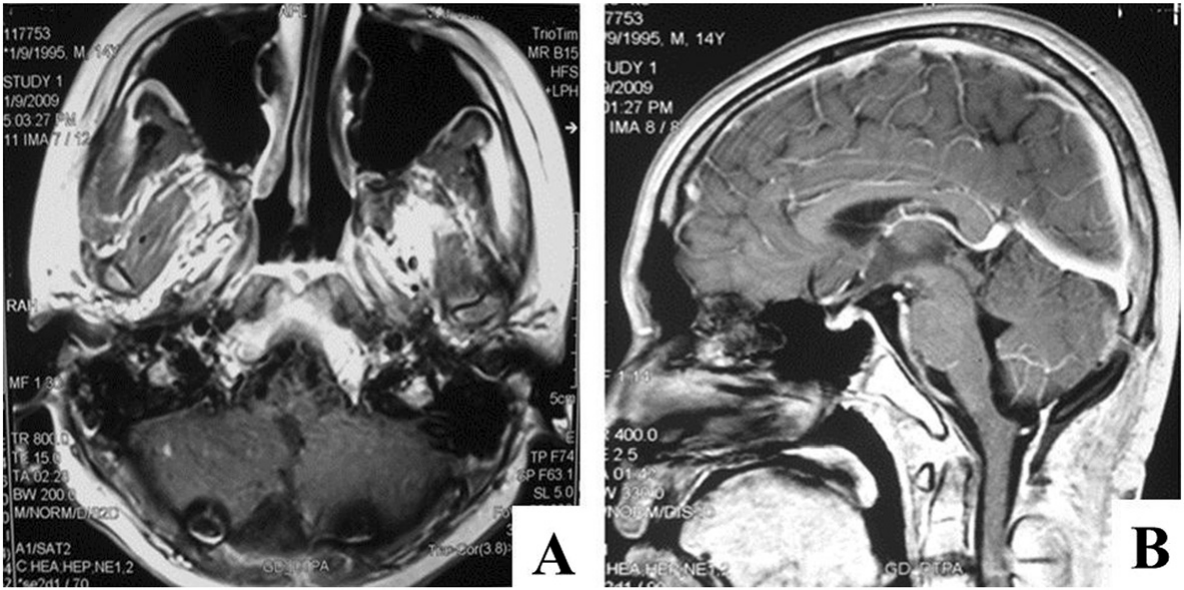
Axonal (A) and sagittal (B) brain magnetic resonance imaging (MRI) scans 6 months after surgery (2 months after chemotherapy and radiotherapy) demonstrating image-complete resection.
